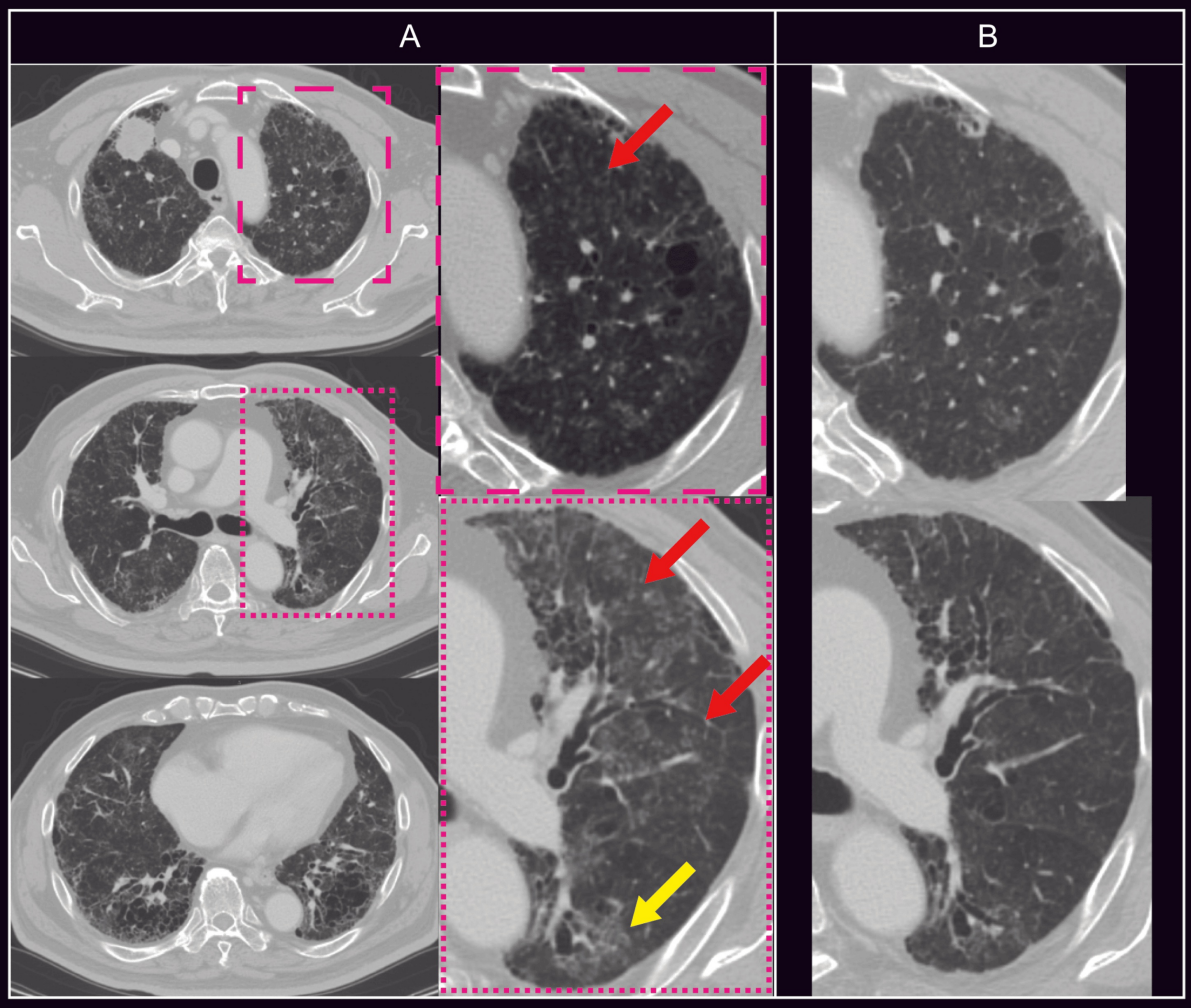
Chest CT obtained three months after UFT/LV plus bevacizumab treatment initiation at the onset of dyspnea (A), and two weeks after oral corticosteroid therapy (B) Following two weeks of oral corticosteroid therapy, the centrilobular nodules (A, red arrows) and ground-glass opacities (A, yellow arrows) showed marked improvement. UFT: tegafur–uracil; LV: leucovorin; OCS: oral corticosteroid therapy
